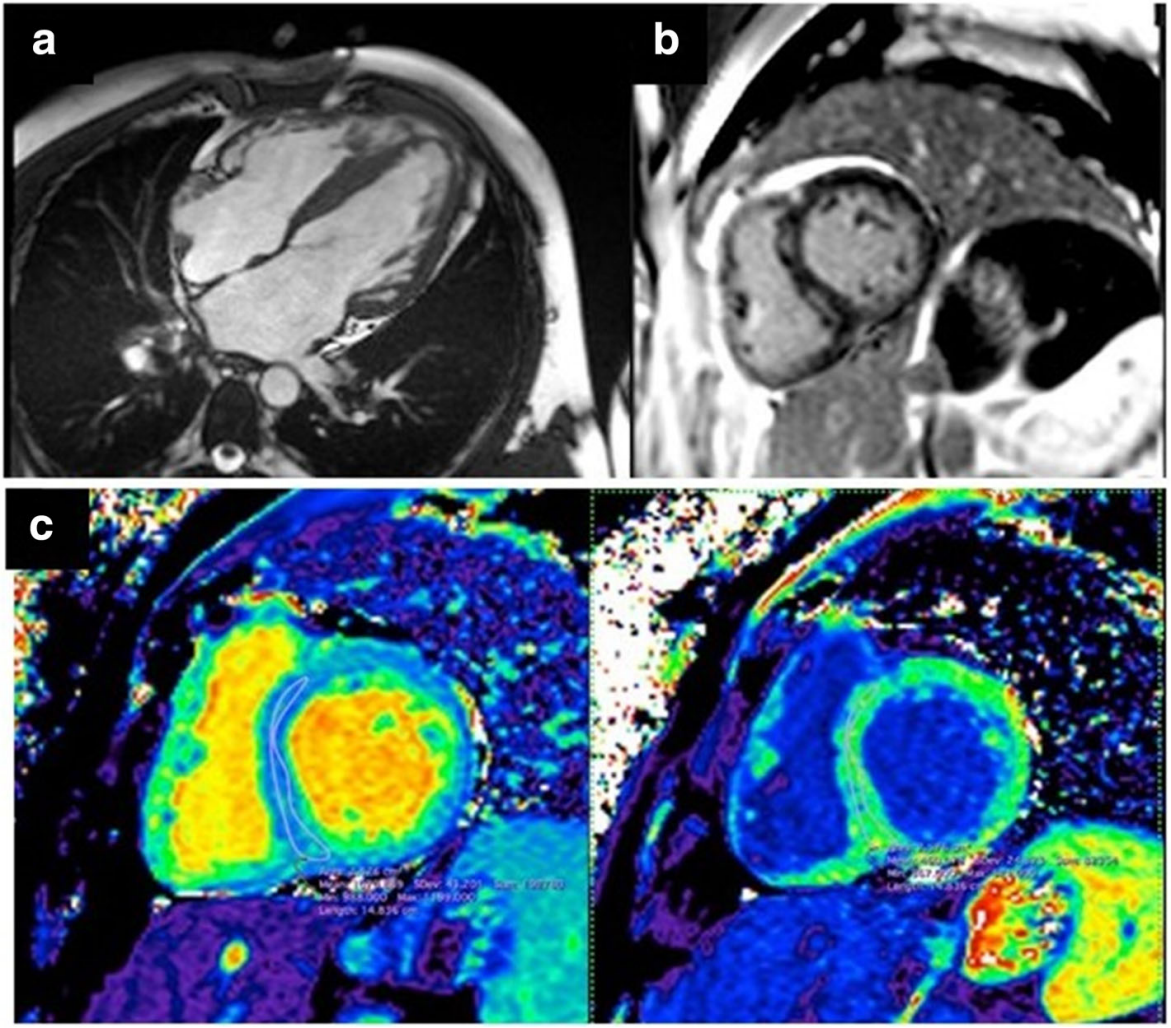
Cardiac MRI. a. Biventricular hypertrophy with maximal wall thickness of 19 mm at inferoseptal level; b. Late gadolinium enhancement basal mid-myocardial disposition. C. Diffuse high T1 values (1074 ms precontrast administration) with extracellular volume expansion to 37%
